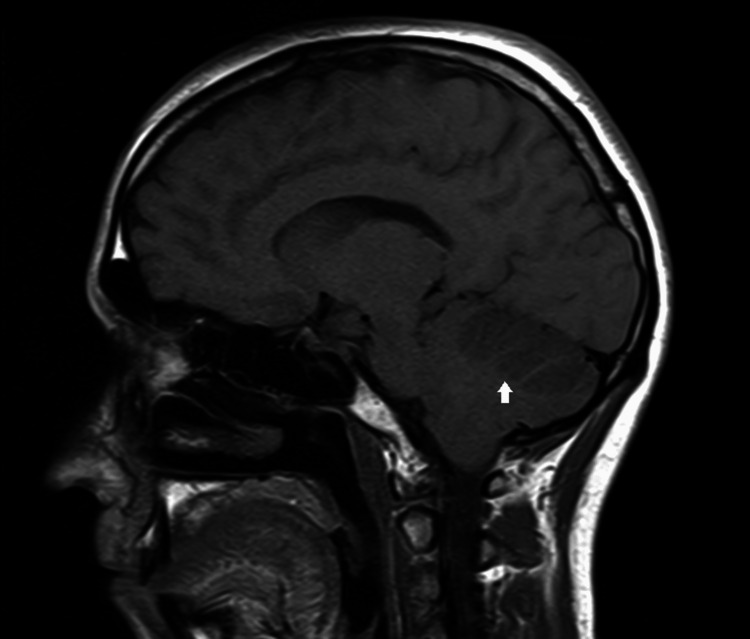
MRI brain (sagittal view, T1) showing right cerebellar ischemic stroke (white arrow)
